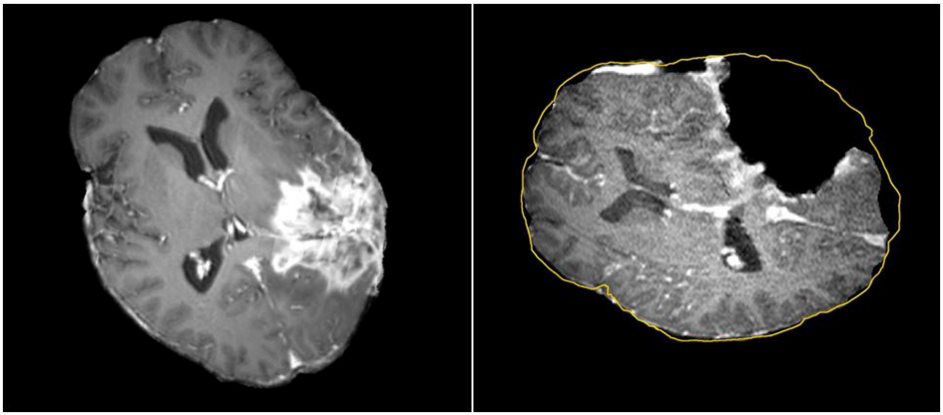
Discrepancies between preoperative and intraoperative MR Imaging before and during neurosurgery. Left: preoperative MRI; Right: intraoperative MRI acquired after a part of the tumor is removed. The yellow outline indicates the preoperative brain outline after a rigid rotation. The large dark cavity is the tumor resection.
